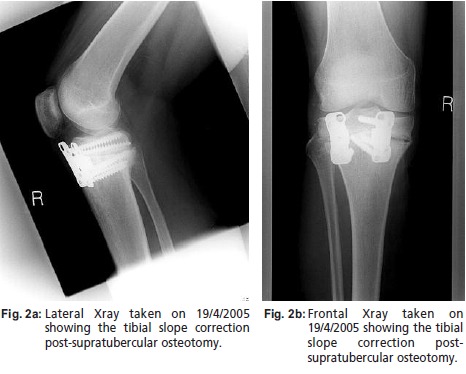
(a)Lateral Xray taken on 19/4/2005
